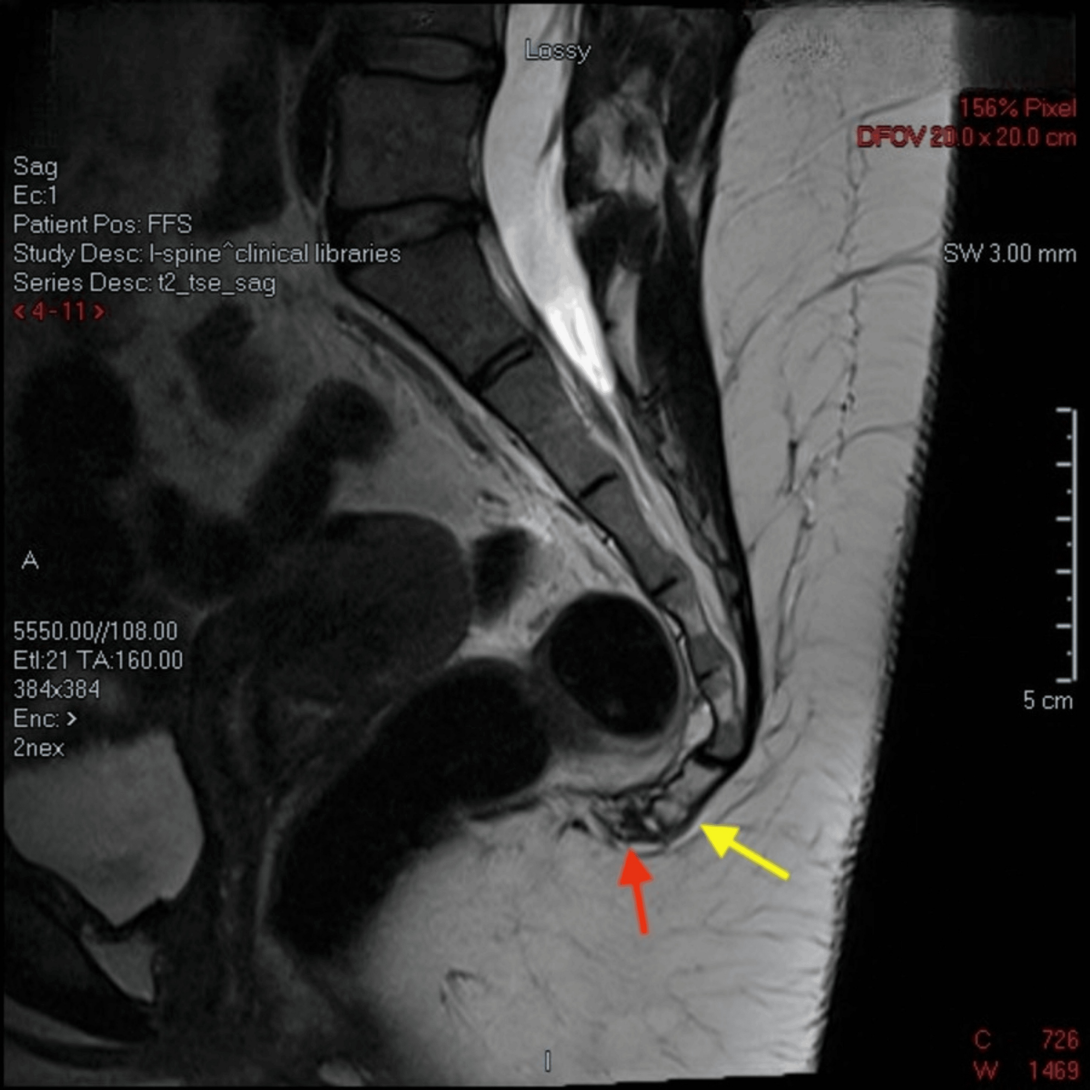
Non-contrast sagittal T2 MRI sequence Yellow arrow indicates transverse, complete, and anteriorly displaced fracture of the Cy2 segment of the coccyx, with fragment traction measuring 3 mm. Red arrow indicates well-demarcated T2 mixed hypo- and hyperintensity in the coccygeal synchondrosis remarkable for early callous bone formation.
